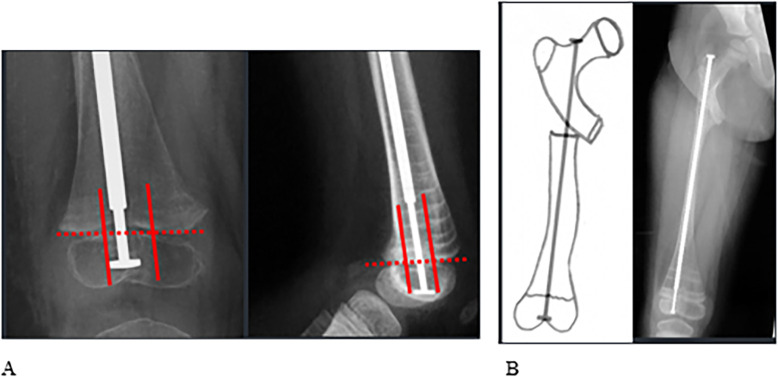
This figure shows optimal positioning of the hardware in the proximal and distal femur. A: In order to define the optimal position of the nail distally, we virtually divided the distal femoral epiphysis into three compartments. A good positioning was defined as the distal end of the male rod passing through the middle compartment of the epiphysis on AP (left) and lateral (right) X-rays. B: Proximally, the position was considered optimal when the female rod exited through the femoral neck.
